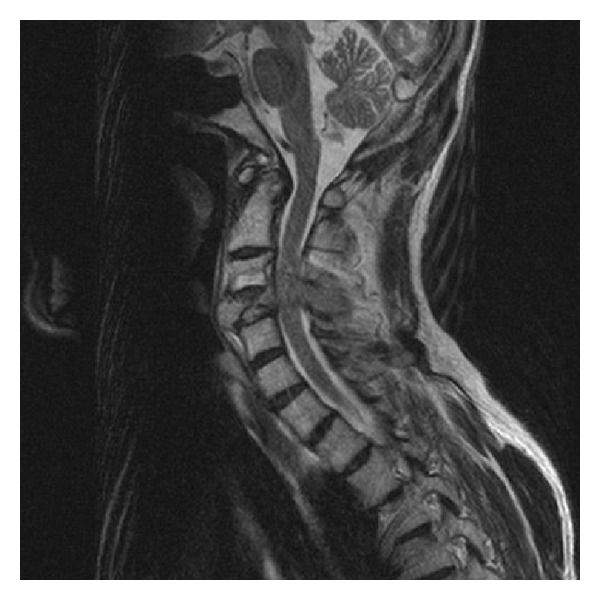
MRI of the cervical spine demonstrating erosive metastatic tumor of the C4 vertebral body.
